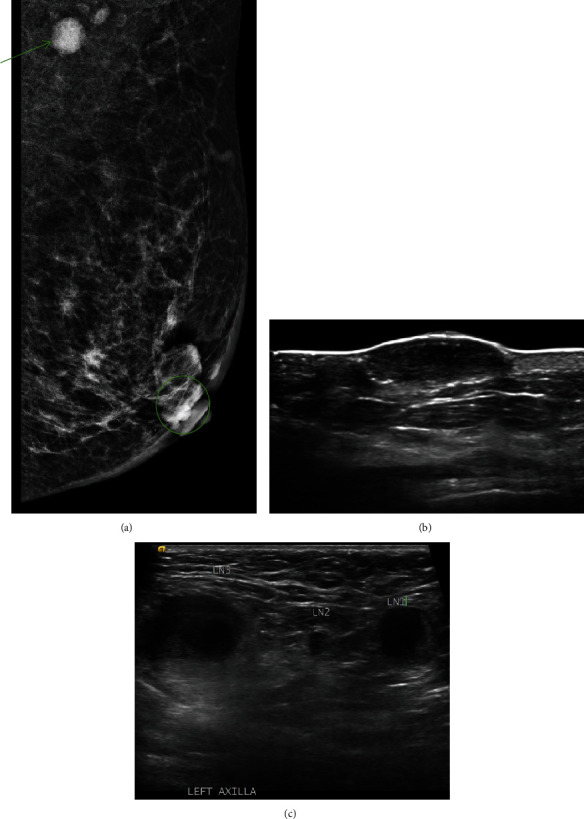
(a) Mediolateral oblique mammogram of the left breast showing a circumscribed periareolar opacity (circle) and dense axillary lymph node (arrow). (b) Superficial hypoechoic nodule on left breast sonography. (c) Lymphadenopathy on left axilla sonography.
